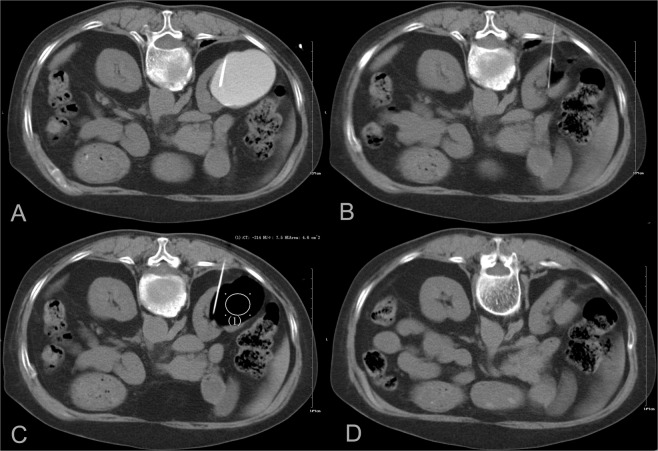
A 74-year-old female with persistent pain in the right loin for 2 months. (A) The cyst was not punctured through renal parenchyma considering that the needle tip was located at the cyst base. (B) During replacement, at least 10–20 mL of liquid was retained in the cyst for the operator to confirm that the needle tip was inside the cyst. At this point, even though the cyst wall collapses, the needle tip near the cyst base remains within the cyst. (C) After completing the second replacement, approximately 75% of the cyst volume of the ethanol was retained in the cyst, with a CT value of −214 HU, indicating an ethanol concentration of >90%, which was retained for 5 min. (D) After the procedure, the cyst wall completely collapsed and the cyst cavity disappeared.
